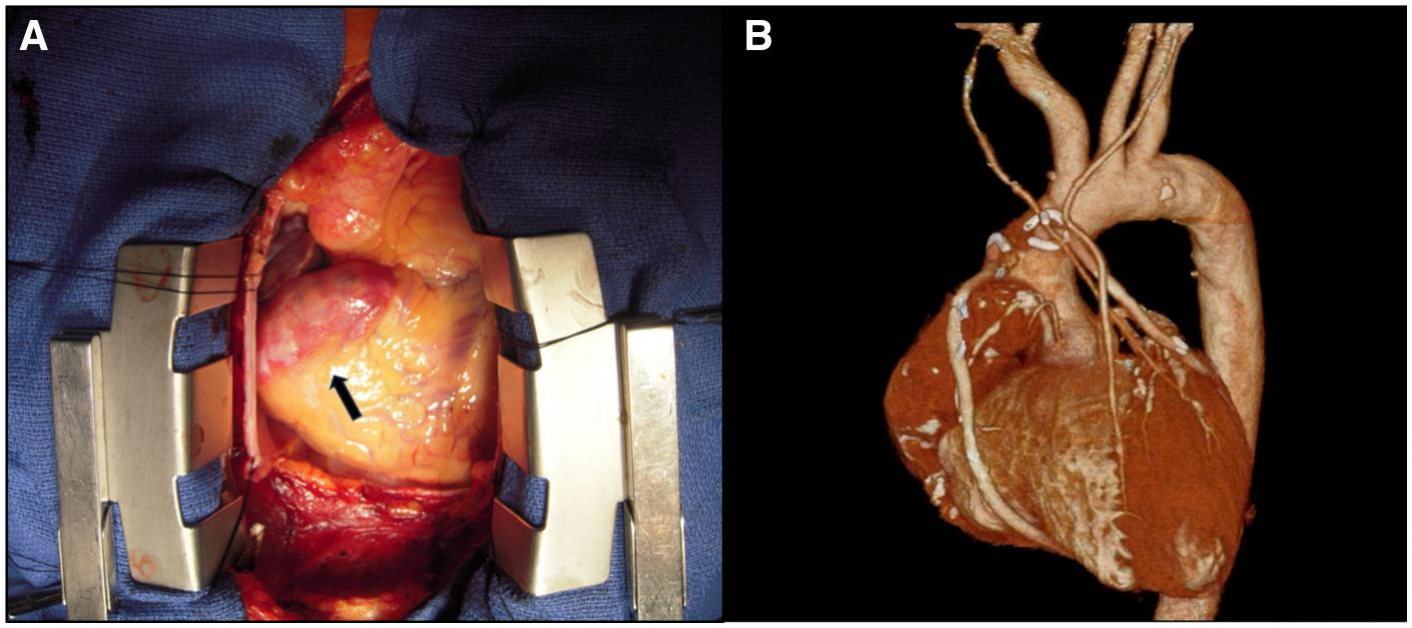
(A) Intraoperative Image Showing a Giant Right Coronary Artery Aneurysm (Arrow). (B) Computed Tomography Finding of the Coronary Artery Bypass Grafting
